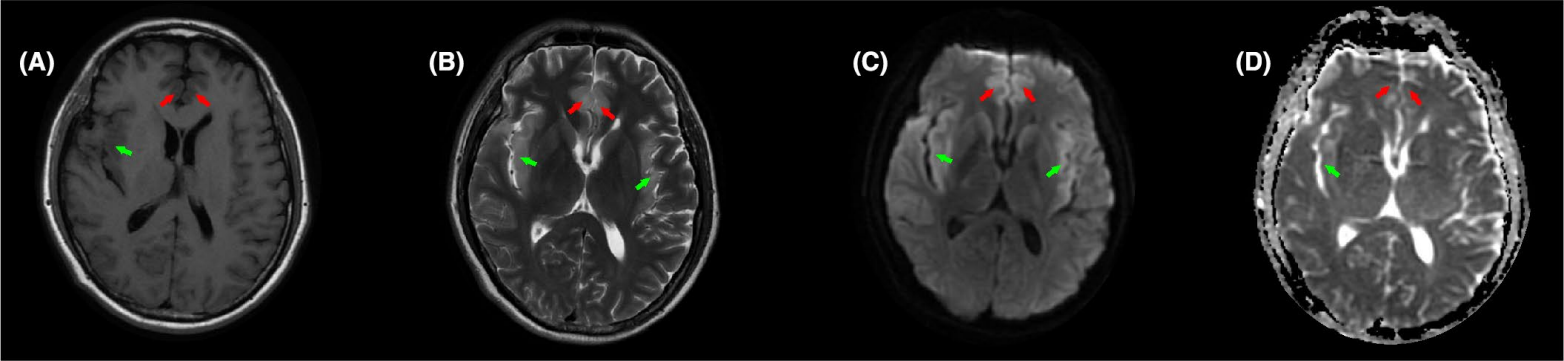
Cranial MRI showed hyperintense signal distributed symmetrically in bilateral frontal lobe (A and B, red arrow) and temporal lobe (A and B, green arrow). Diffusion‐weighted MRI showed hyperintense signal distributed symmetrically in bilateral frontal lobe (C, red arrow) and temporal lobe (C, green arrow). Apparent diffusion coefficient MRI showed hypointense signal distributed in bilateral frontal lobe (D, red arrow), and right temporal lobe (D, green arrow)
